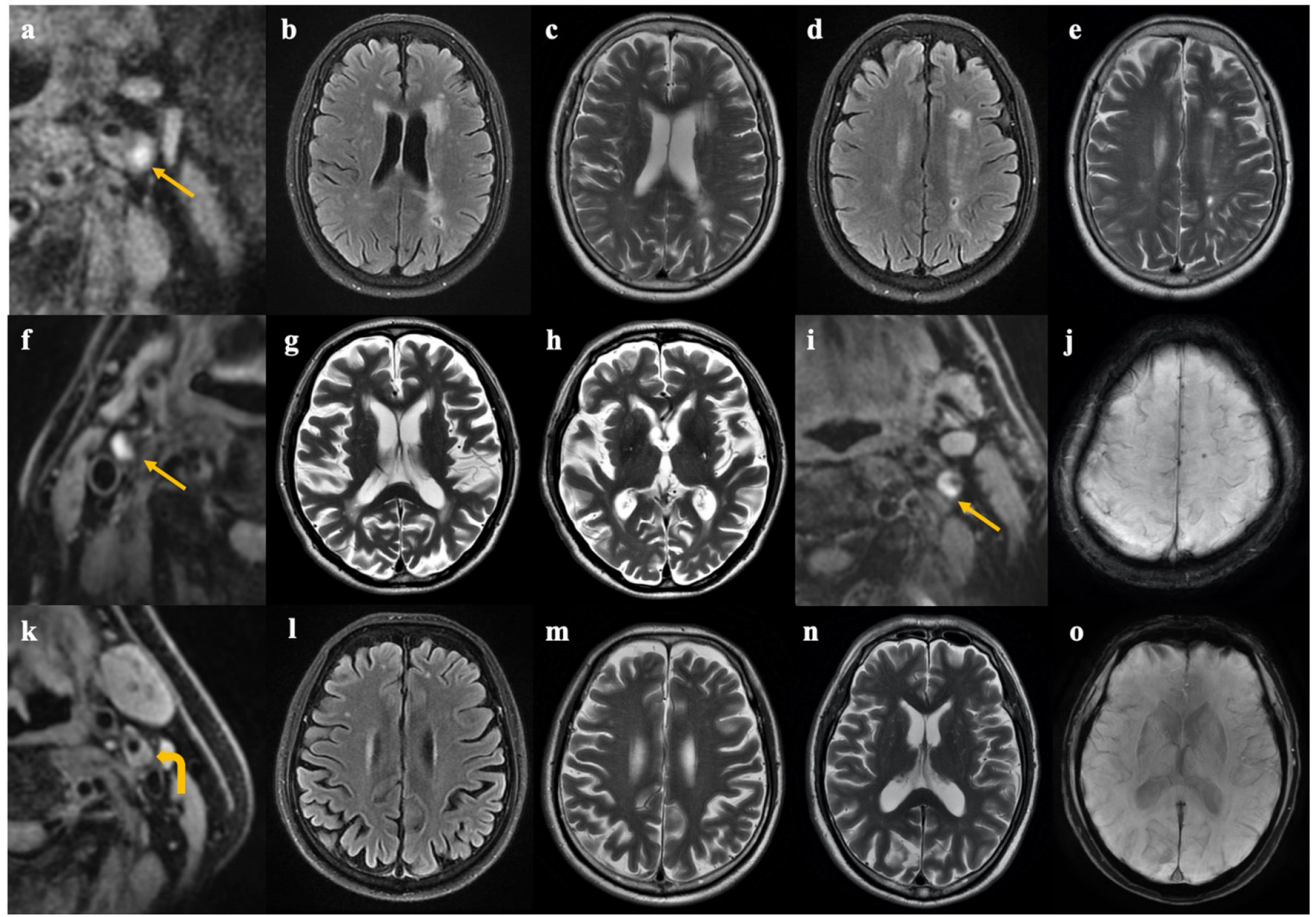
Representative cases of patients with and without unilateral carotid IPH. Case 1 (a–e). A 68-year-old female complained of weakness of right limbs, and high-resolution MRI for carotid plaque showed carotid intraplaque hemorrhage (IPH) on the left side (a). Brain MRI showed more severe white matter hyperintensities and lacunes on the left hemisphere than that on the contralateral hemisphere (b–e). Case 2 (f–h). An 82-year-old male complained of transient weakness of lower limbs. High-resolution MRI for carotid plaque showed IPH on the right side (f). Brain MRI showed category-1 perivascular spaces (PVSs) in the left basal ganglia and category-2 PVSs in the right basal ganglia (g,h). Case 3 (i,j). An 85-year-old male complained of dizziness for 1 month. High-resolution MRI for carotid plaque showed IPH on the left side (i), and a cerebral microbleed lesion was found on the ipsilateral hemisphere (j). Case 4 (k–o). A 71-year-old male with asymptomatic carotid stenosis. Carotid plaque without hemorrhage on the left side was shown on carotid MRI (k), and cerebral small vessel disease was symmetrical between two hemispheres (l–o). Arrow indicates carotid IPH, and curved arrow indicates carotid non-IPH.
